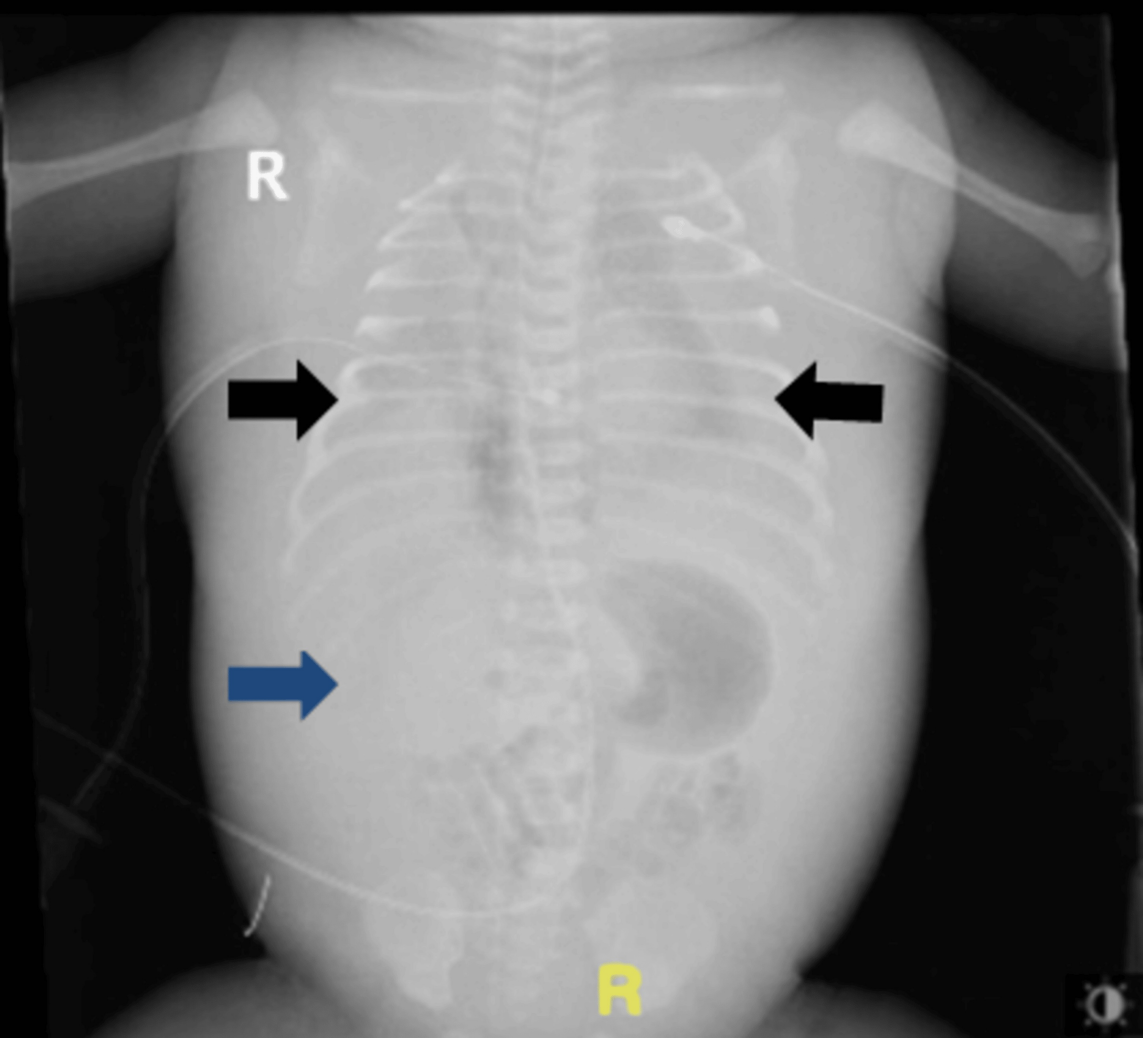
Abdominal and chest X-ray of a neonate with congenital nephrotic syndrome showing bilateral pleural effusion (black arrows) and abdominal ascites (blue arrow).
